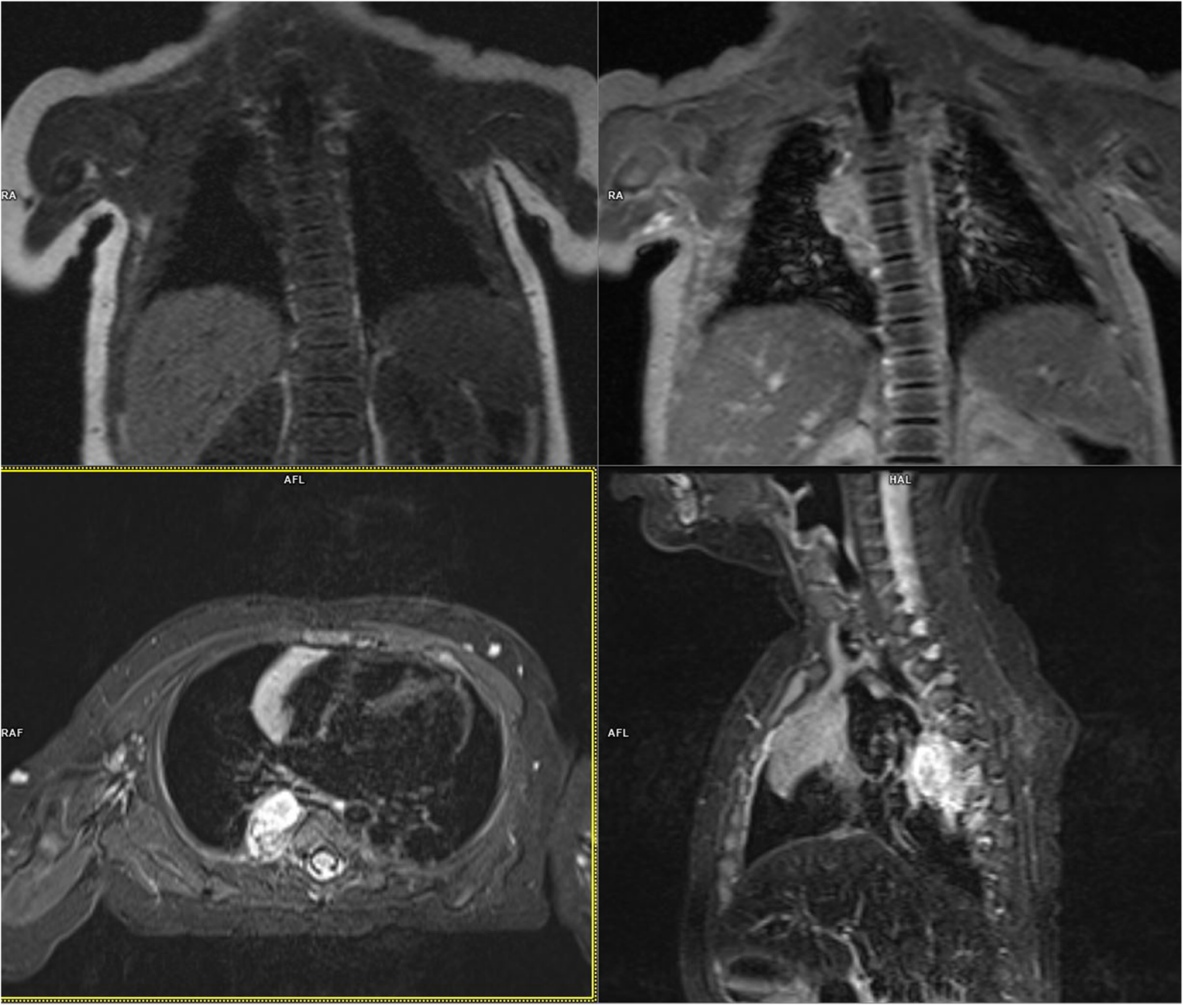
MRI of a boy with a tumor localized in back mediastinum
